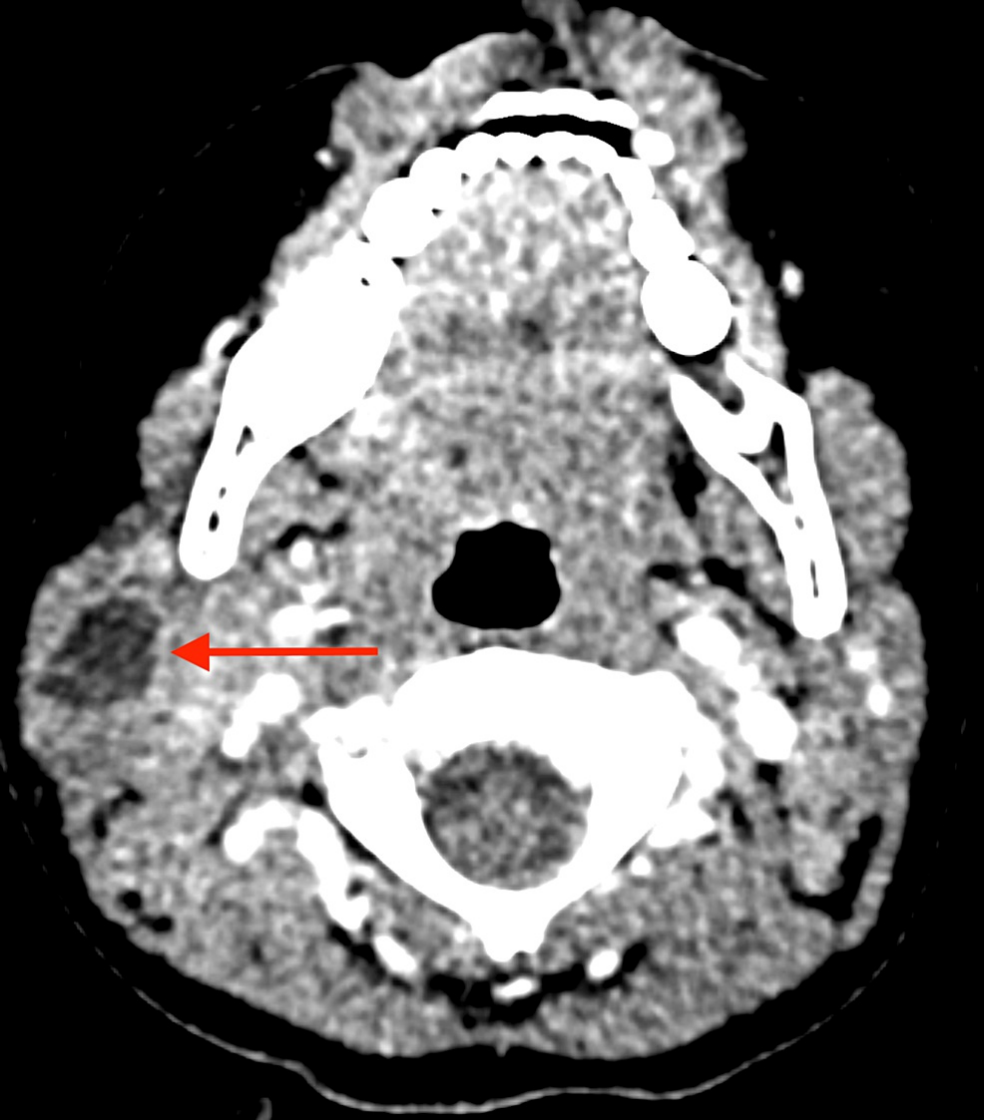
Axial CT view of the right parotid abscess
